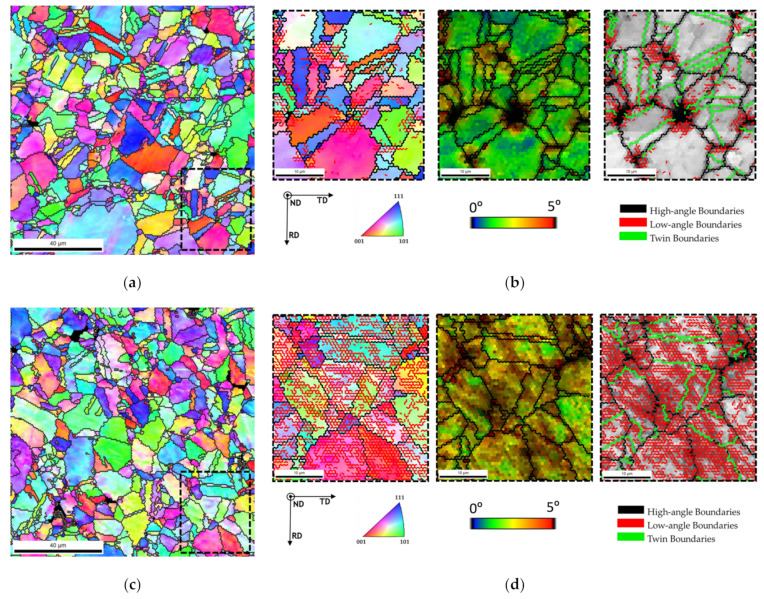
IPF maps of (a) Ni and (c) Ni-CNT produced by ultrasonication. IPF, KAM, and IQ maps with high- and low-angle boundaries (HAGBs and LAGBs) delimited by the (b) area marked in (a,d) area marked in (c).
